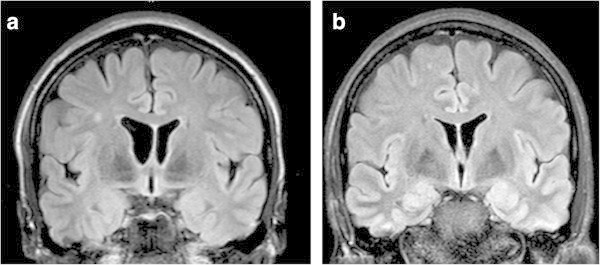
Coronar brain MRI slices (FLAIR), in (a), on the left side, with one WML in a responder and in (b), on the right side, with three WML in a non-responder.
